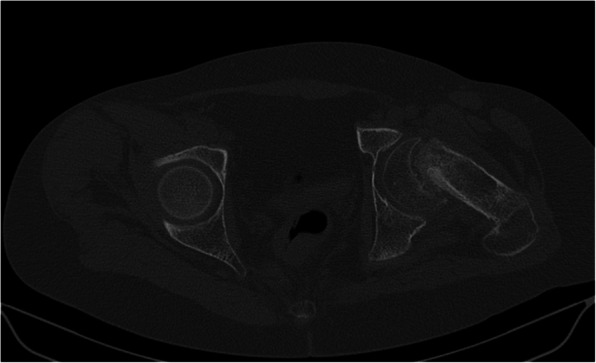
SCFE in transverse plane CT before surgery
